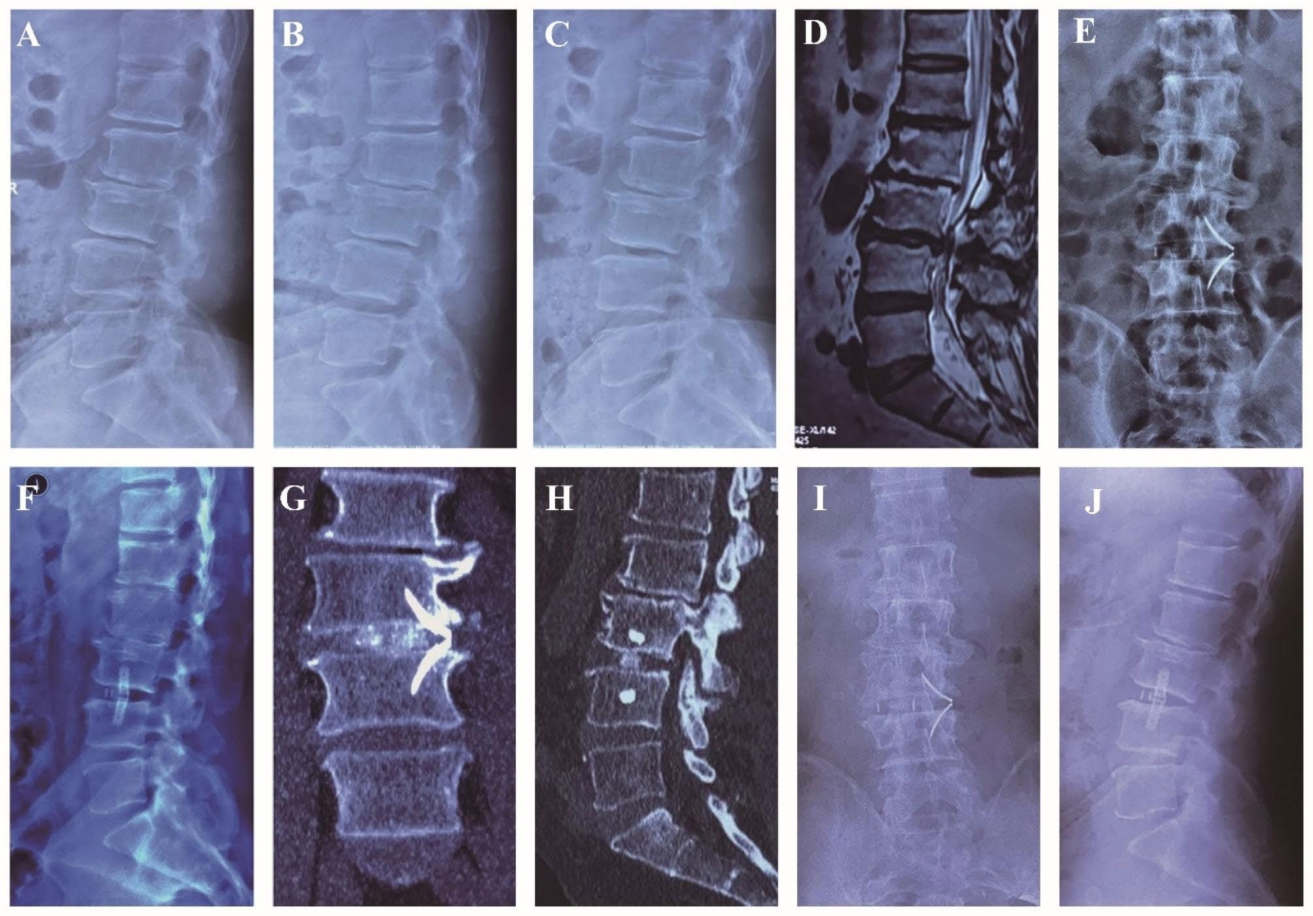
Pre-, Post-operative and follow-up images obtained in a 70-year-old male who had low back pain that exacerbated during activity, and was diagnosed with L3-4 degenerative spondylolisthesis. The symptoms disappeared after SA-LLIF. (A) The lateral X-ray shows lumbar spondylolisthesis of grade I; (B) The flexion X-ray shows reduction of spondylolisthesis; (C) The extension X-ray shows exacerbation of spondylolisthesis; (D) MRI shows L3-4 intervertebral disc degeneration and herniation; (E F) postoperative AP and lateral radiographs shows that cage was located in the center of the intervertebral space and good sagittal alignment was achieved; (G, H) CT coronal/sagittal position shows good position of cage, plate and bone graft; (I, J) 3 months after operation, the AP and lateral X-ray shows the plate and cage were stable
